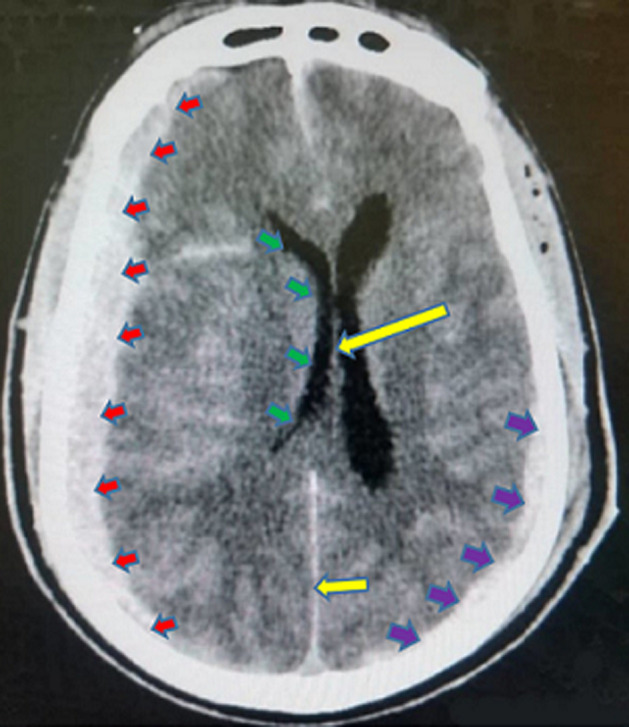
a non-contrast head CT scan showing acute subdural hematomas with loss of gyri and sulci (red short and violet arrows), mass effect (green short arrows), midline shift (yellow arrow) compared to the normal midline position (yellow short arrow)
